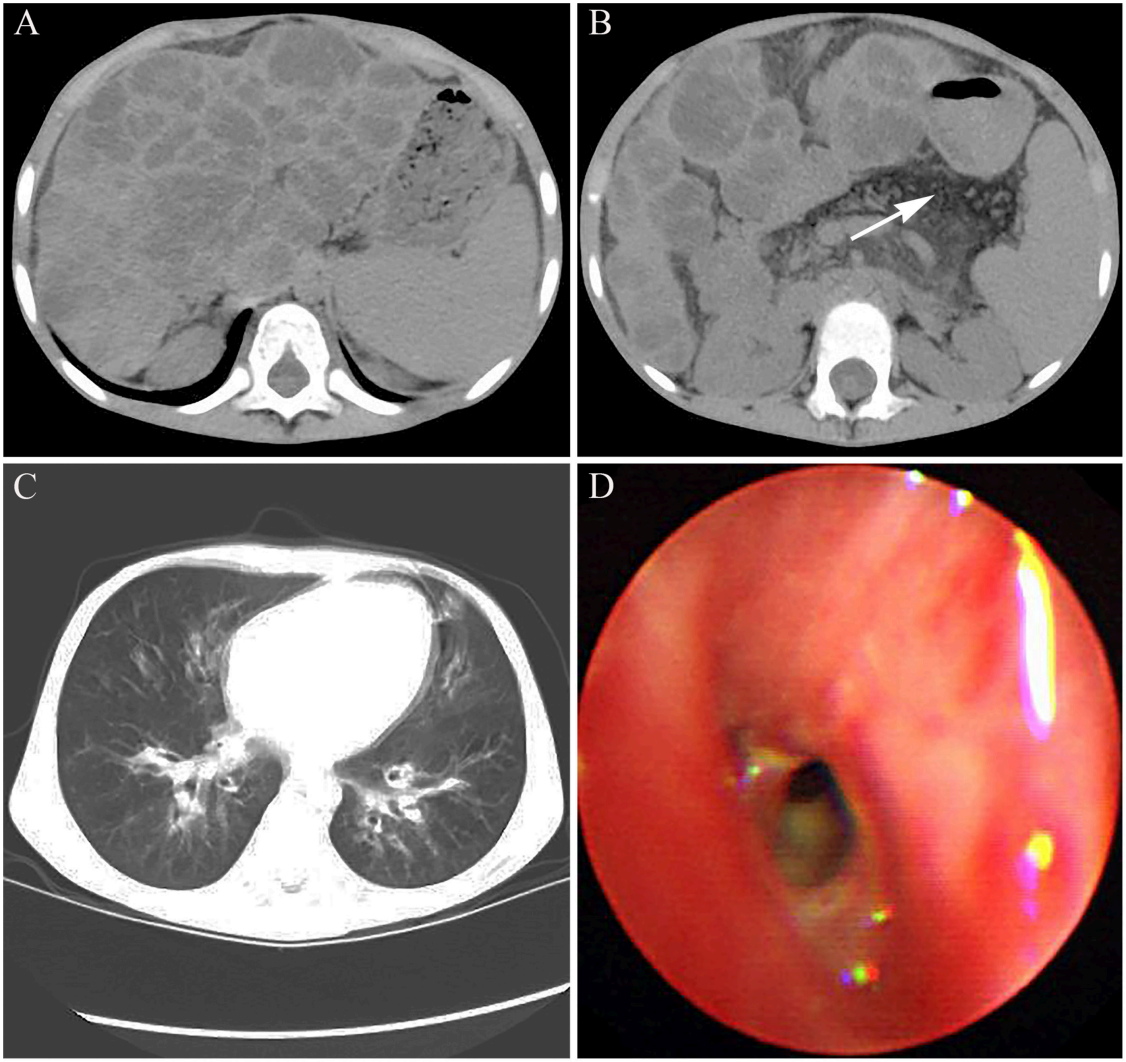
The images of CT and bronchoscopy in 2016. (A) enlarged spleen and macronodular cirrhosis on the basis of diffuse hepatic steatosis and (B) fatty replacement of the atrophied pancreas indicated by white arrow were observed. (C) Mild bilateral bronchiectasis and (D) purulent secretions were observed.
